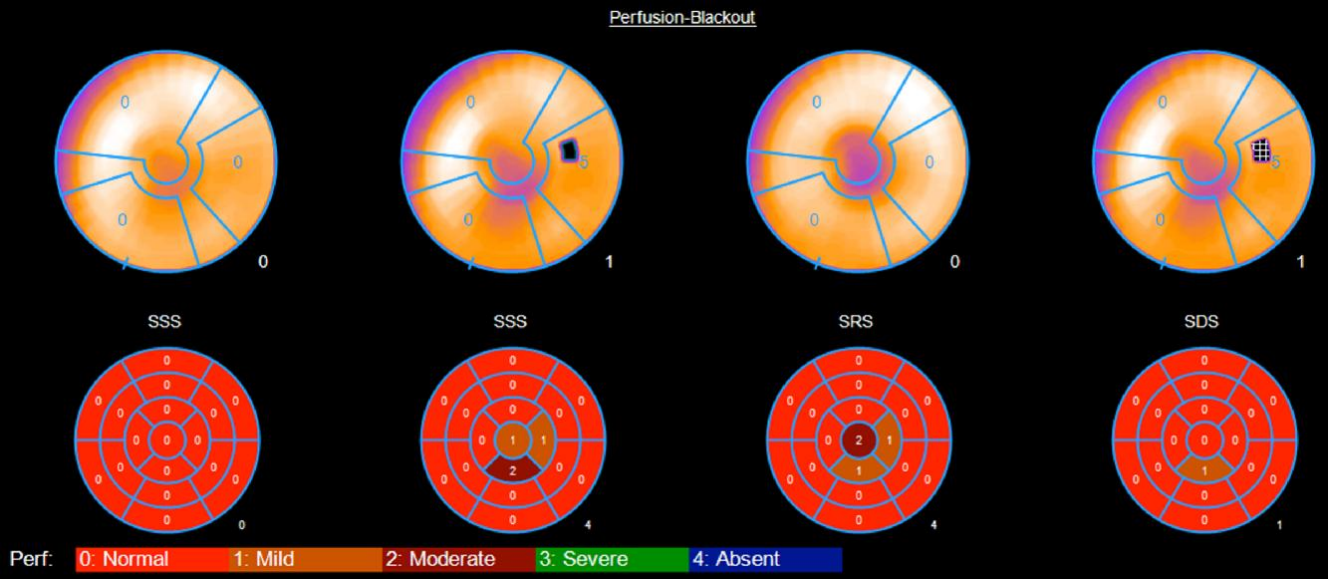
Single photon emission computed tomography 17-segment model for Case 2, showing no significant ischaemia and thus test-negative.
